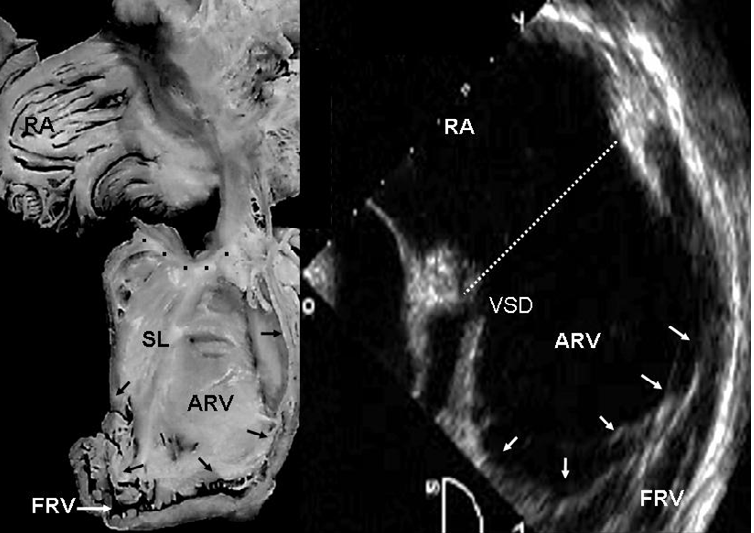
Internal view of cardiac right chambers show extreme (grade III) leaflet tethering to the ventricular wall (arrows). There is severe reduction of the functional portion of the right ventricle, the apical portion of which is tunnel-shaped chamber. The echocardiographic image shows the great predominance of the atrialized portion of the right ventricle and a perimembranous ventricular septal defect. VSD: Ventricular septal defect. Other abbreviations as before.
